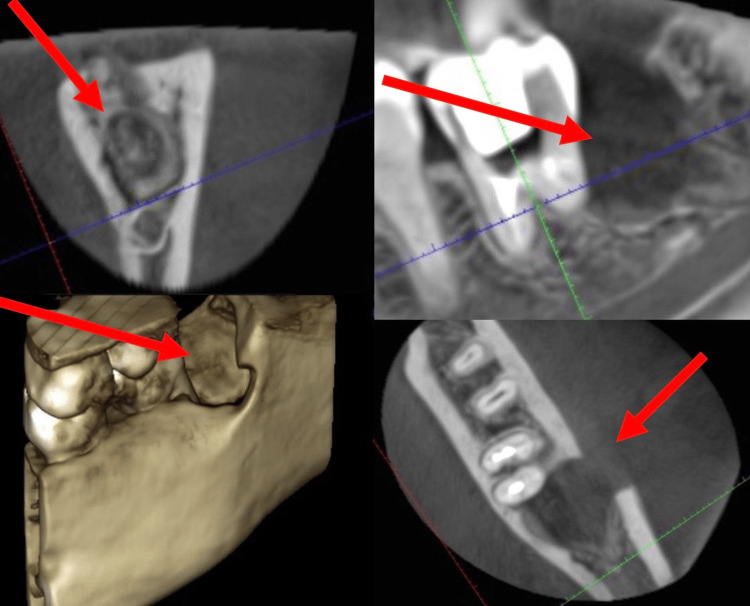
Cone beam computed tomography of the extracted site.
